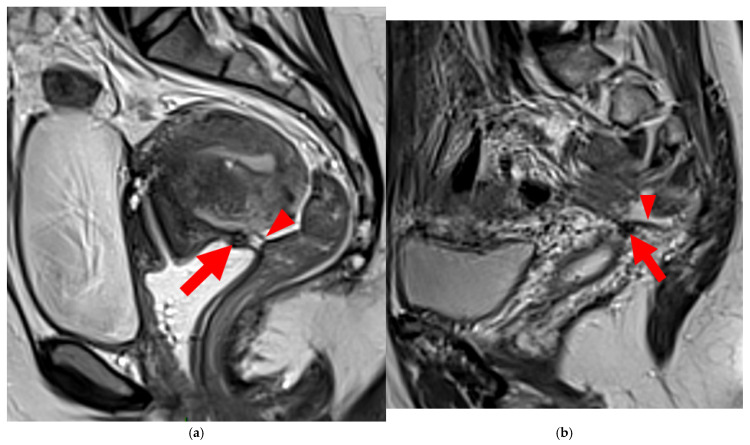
Pelvic MRI scans of two patients with HTD type 5A USLs. (a,b) Sagittal T2WI: nodularity with regular margins (*arrows*) within the right USL (*arrowheads*).
